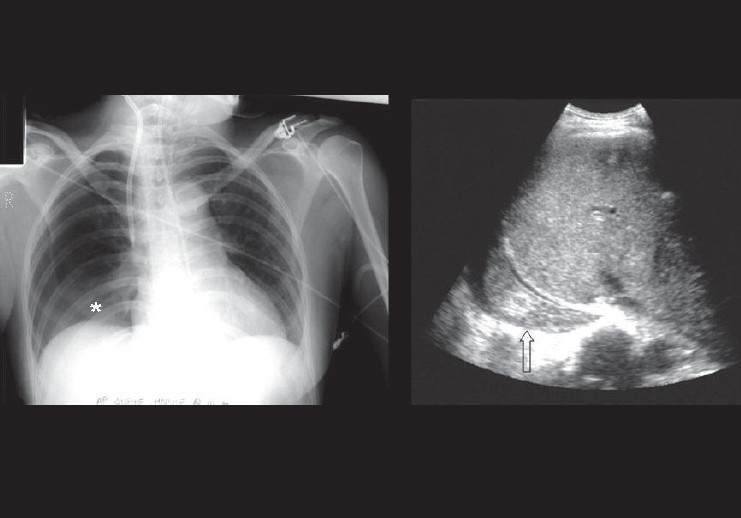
Frontal radiograph showing vague opacification at the left lung base, suggestive of a pleural effusion that followed a difficult intravenous line placement. The ultrasound image (right) shows solid component within the posterior costophrenic angle, suggestive of a hemothorax. An ultrasound scan can easily differentiate a clear pleural effusion from a hemorrhagic pleural effusion
